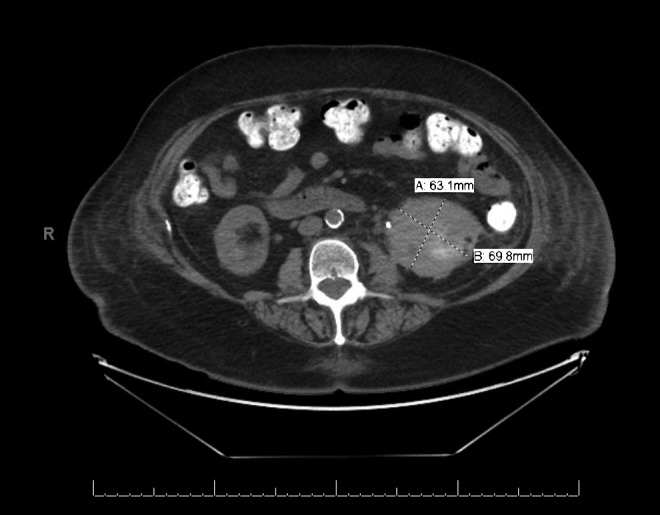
Abdominal CT scan at presentation.
